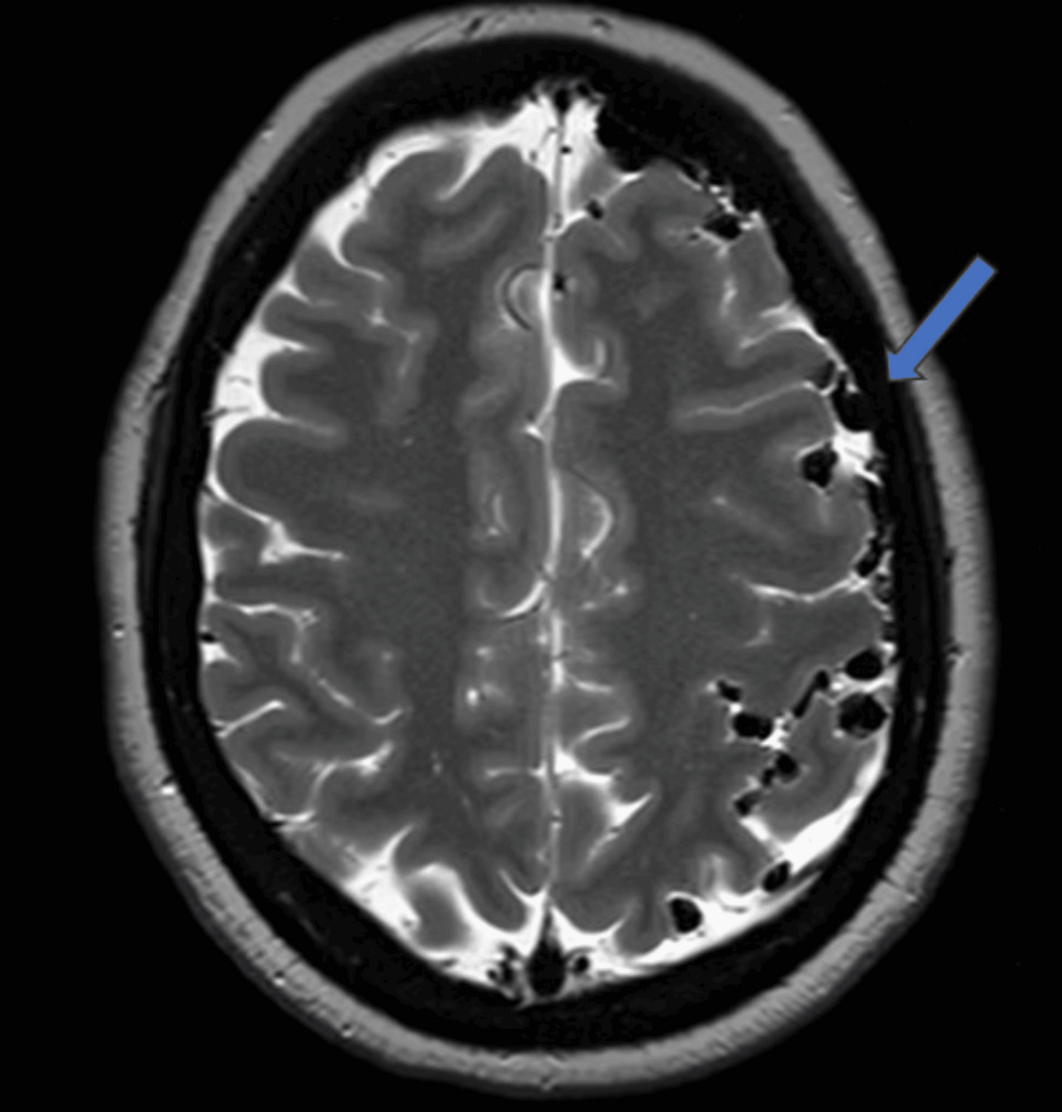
MRI brain axial T2W image at the level of the frontoparietal lobes The image shows extensive air foci (blue arrow) along the subarachnoid spaces of the brain, more on the left side.
